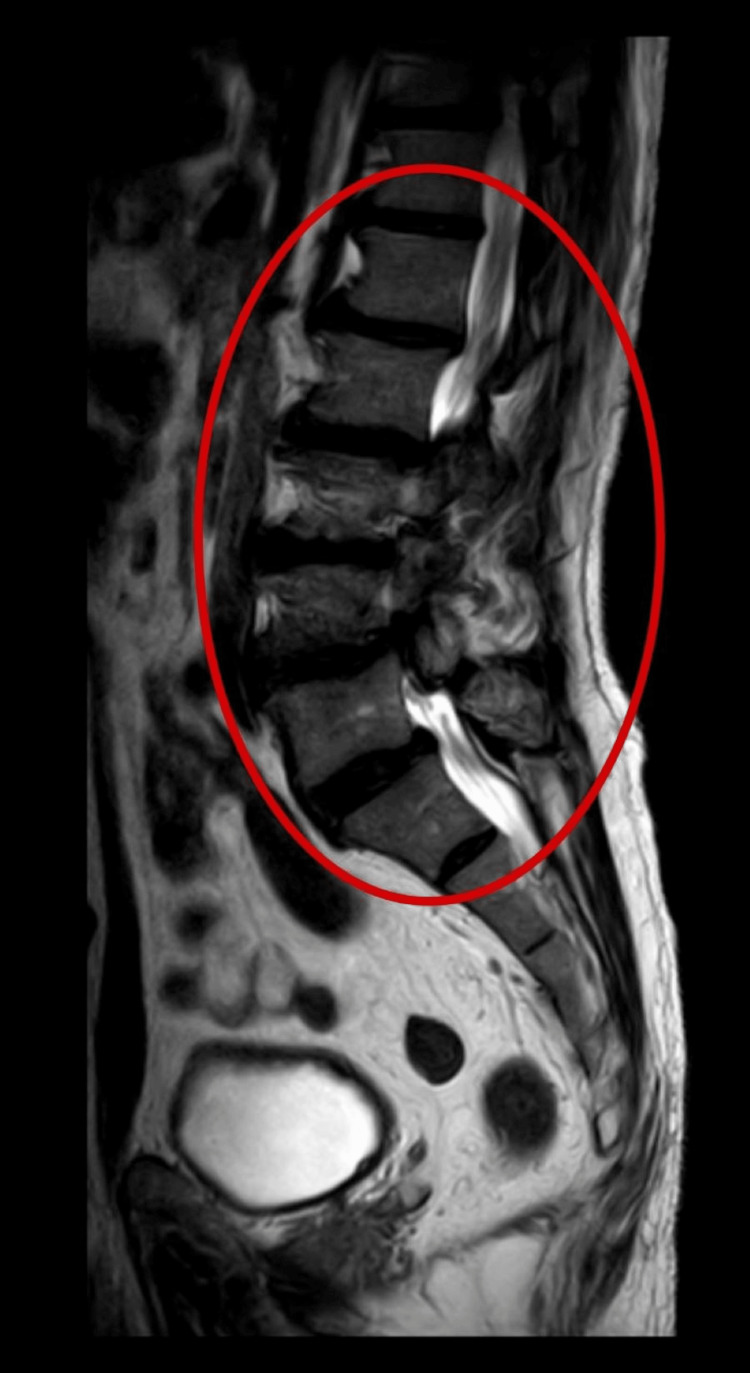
Pre-operative MRI showing compression at L1-L5 (red circle)
